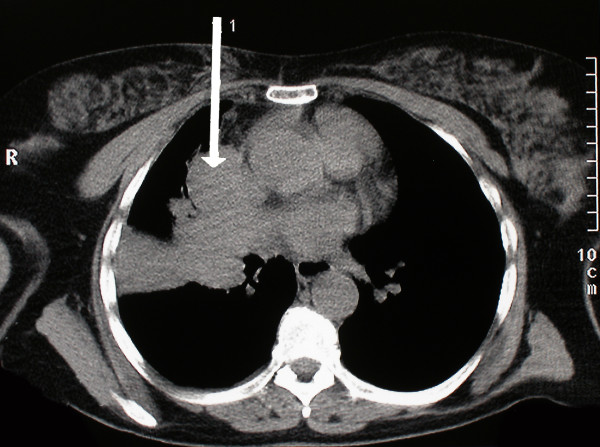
Axial CT image demonstrating the right hilar mass extending into the right middle lobe and distorting the outline of the right and left atria with CT guided percutanous biopsy of the mass.
